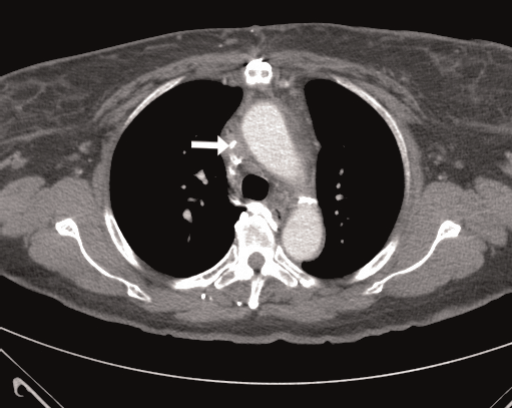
CT scan with contrast of the chest shows narrowing of the SVC.
